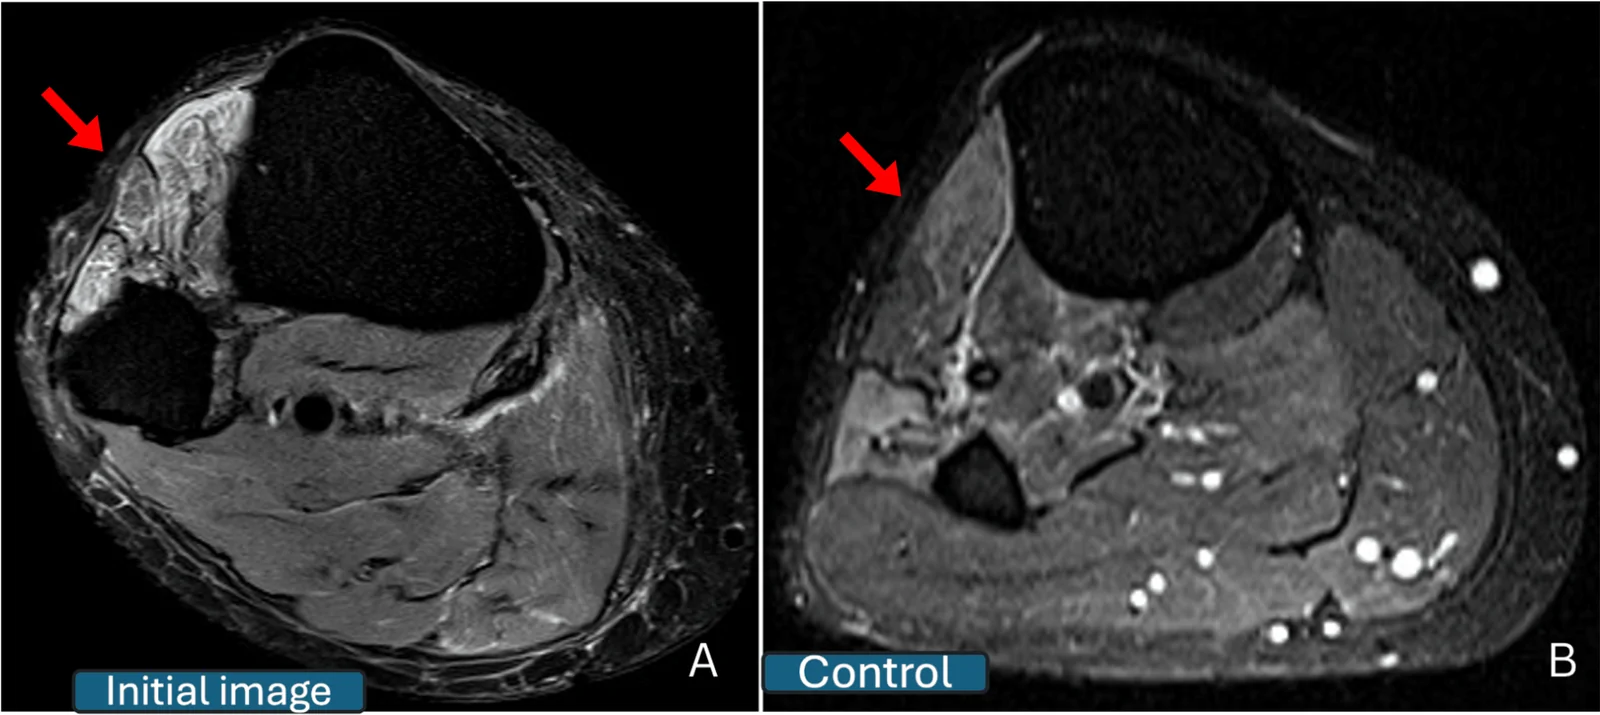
Serial axial MRI of the right leg demonstrating denervation changes A: Axial PD sequence with fat saturation. Corresponds to the initial study, showing muscle edema in the anterolateral compartment of the leg (including the peroneus longus, extensor digitorum longus, and tibialis anterior) due to acute denervation. B: Axial STIR sequence. Follow-up study demonstrating persistent mild muscle edema in the peroneus longus with marked atrophic changes, consistent with subacute/chronic denervation.
